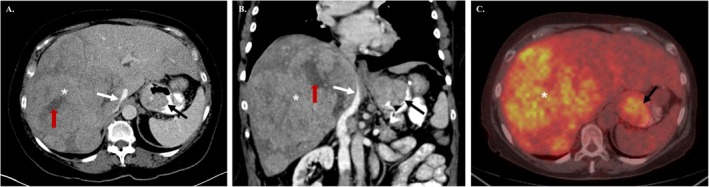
Gastric hepatoid adenocarcinoma with liver metastases in a 65‐year‐old‐female with prior history of Roux‐en‐Y gastric bypass surgery. Contrast enhanced axial CT image (A) and coronal reformatted image (B) showing a large lobulated and heterogeneously enhancing metastases in right lobe liver (*) with mass effect on inferior vena cava (white arrows) and areas of necrosis (red arrows). The primary HAC is identified in the gastric pouch (black arrows). Fused fluorodeoxyglucose (FDG) positron emission tomography (PET)‐CT image (C) showing increased tracer uptake in the hepatic metastasis (*) and in the primary gastric tumor (black arrow).
